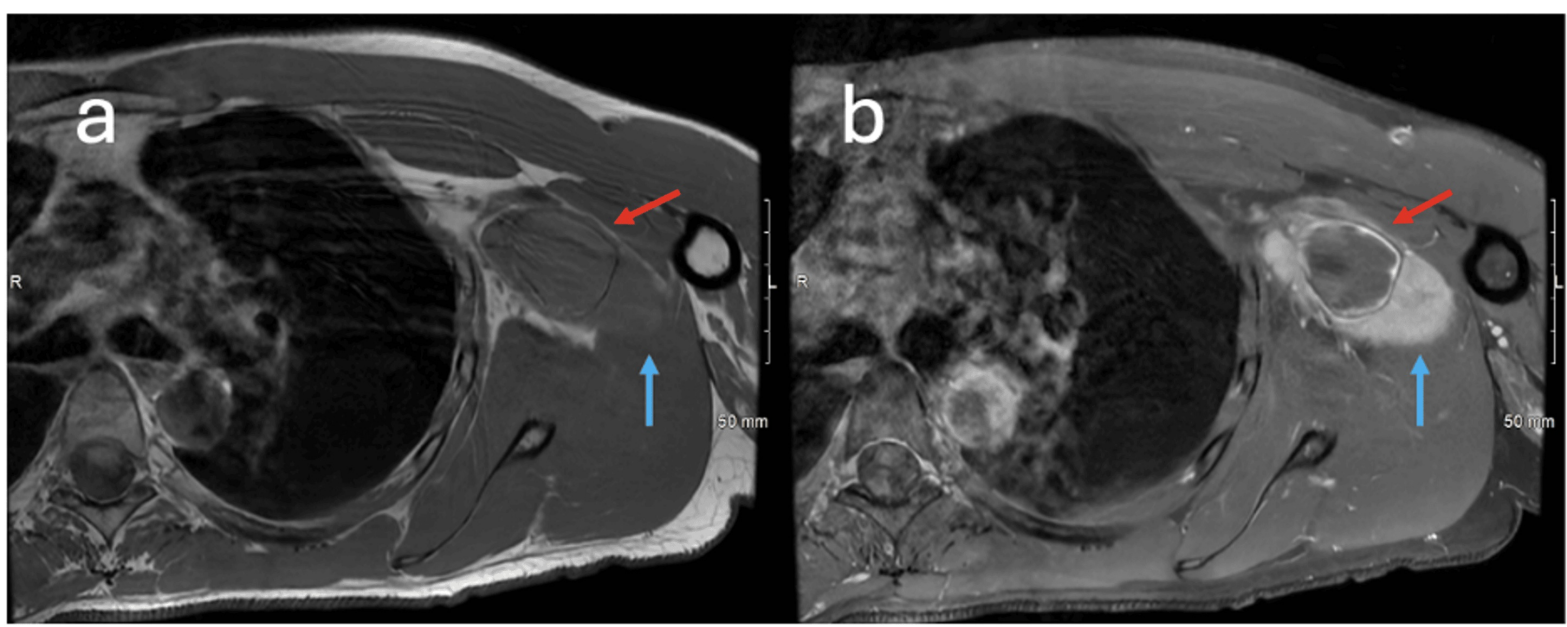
Axial T1 and T1 fat-saturated post-contrast MRI of the left brachial plexus demonstrating cystic and solid lesions Axial T1 image of the left brachial plexus (a) demonstrates a complex T1 dark cystic (red arrow) and a T1 isointense to muscle (blue arrow) lesion in the region of the left brachial plexus. Axial T1 fat-saturated post-contrast image of the same level (b) demonstrates faint peripheral enhancement of the cystic lesion (red arrow) and solid enhancement of the adjacent solid lesion (blue arrow).
